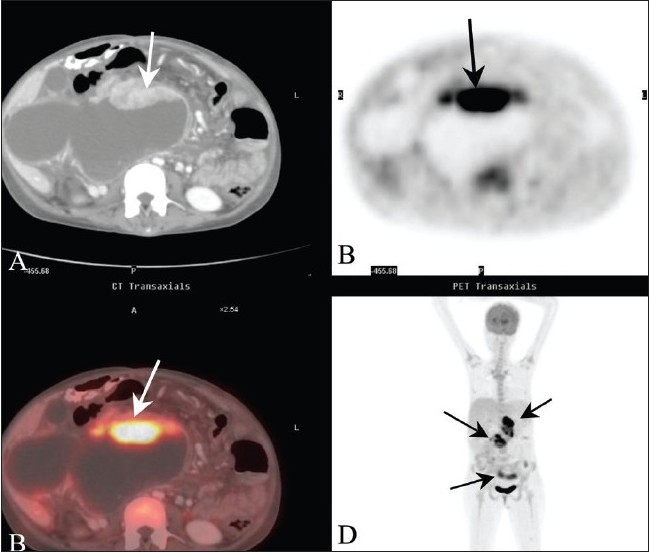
(A-D): Contrast-enhanced CT (A) shows the soft tissue mass (arrow) in the anterior aspect of the cyst, with increased uptake (arrow) in the FDG PET (B) and PET/CT fusion (C) images. The coronal PET image (D) shows additional areas of increased uptake (arrows) in the pancreatic head, pelvis, and left lower quadrant
